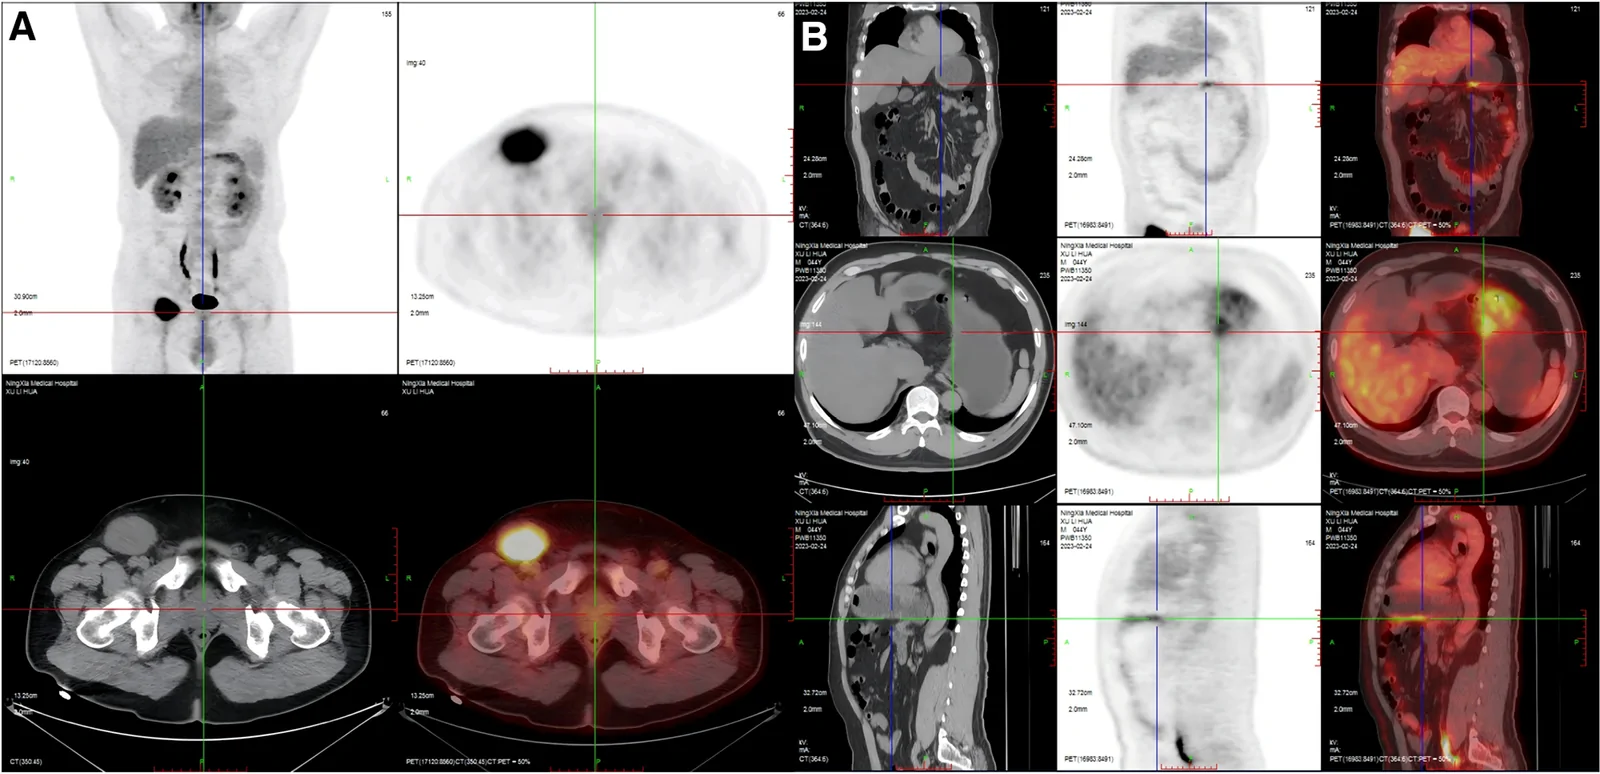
18F-FDG PET/CT scan performed on February 23, 2024. (A) Intense FDG uptake in the right inguinal lymph node, measuring 5.5 cm in maximum diameter with SUVmax 9.8. (B) Focal mild metabolic activity along the lesser curvature of the gastric body (SUVmax 4.1); subsequent gastrointestinal endoscopy revealed no malignant lesions. No other hypermetabolic foci suggestive of malignancy were identified. CT = computed tomography, FDG = fluorodeoxyglucose, PET = positron emission tomography.
